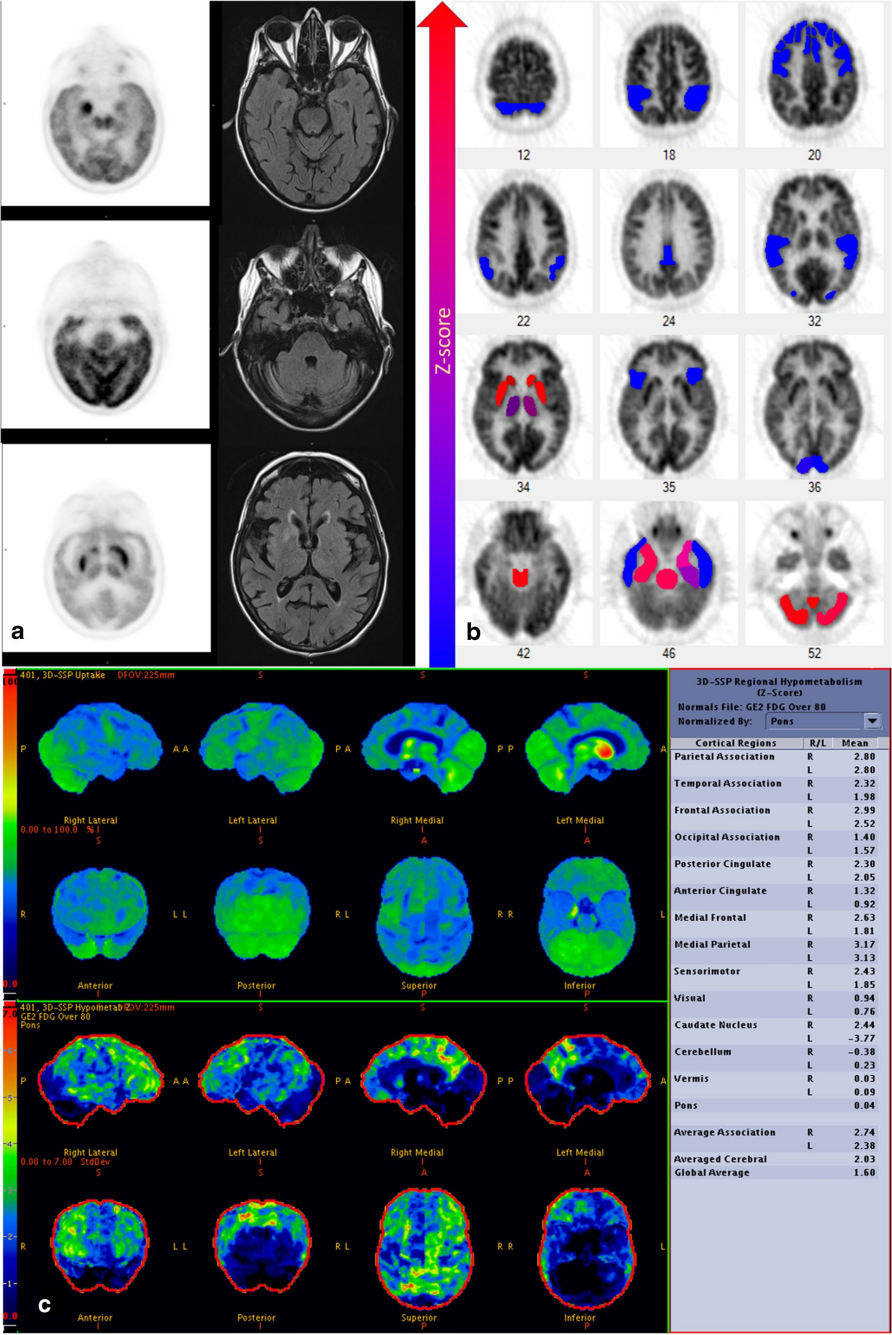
Brain MRI and brain 18F-FDG-PET in a patient with anti-LGI1 autoimmune encephalitis. a MRI images show FLAIR hyperintense foci corresponding to areas of high FDG avidity on PET images in the bilateral basal ganglia, and right medial temporal lobe. In addition, there is symmetric high FDG avidity of bilateral cerebellum, and brain stem. There is mild cortical expansion of the right medial temporal lobe. b NeuroQ™ output with abnormal hypermetabolic regions including the bilateral basal ganglia (slice 34), medial temporal lobes (slice 46), brain stem (slice 46), and cerebellum (slice 52). c CortexID™ output that did not detect the abnormal uptake in the medial temporal lobes and cerebellum. CortexID™ output showed right caudate hypo and left caudate hypermetabolism. The positive Z score values above 1.65 in CortexID^TM^ demonstrate hypometabolism
